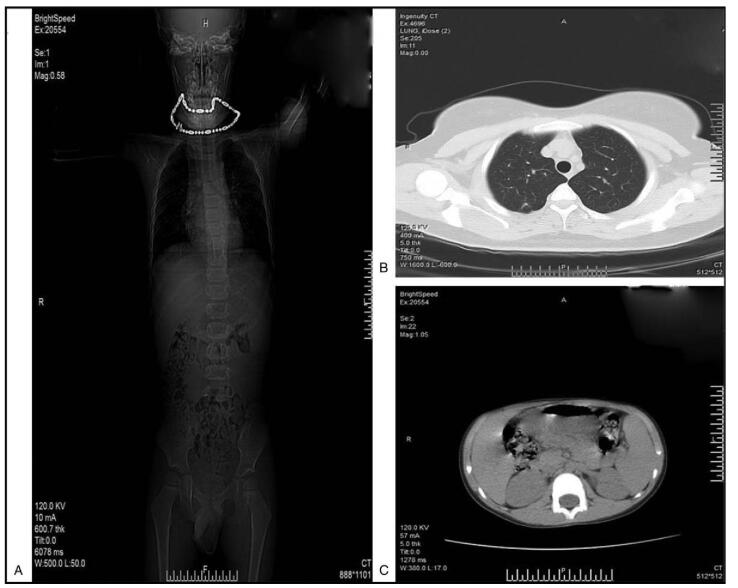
Computed tomography (CT) imaging of patients diagnosed with PMYST after surgery and postoperative chemotherapy (Case 1). (A) Computed tomography scan on admission. (B) Computed tomography scan of the thorax. C. Computed tomography scan of the pelvic cavity.
